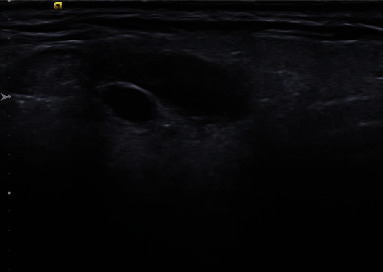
Ultrasound of the right parotid revealing residual hypoechoic/cystic lesion which was subsequently aspirated.
